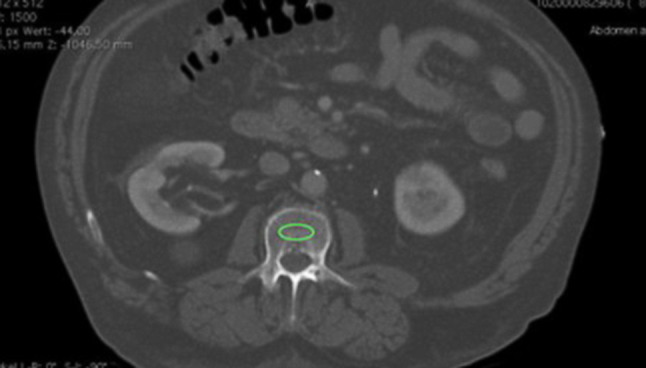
Cross-sectional X-ray image showing measurement of BMD at L1 (marked *green*)
